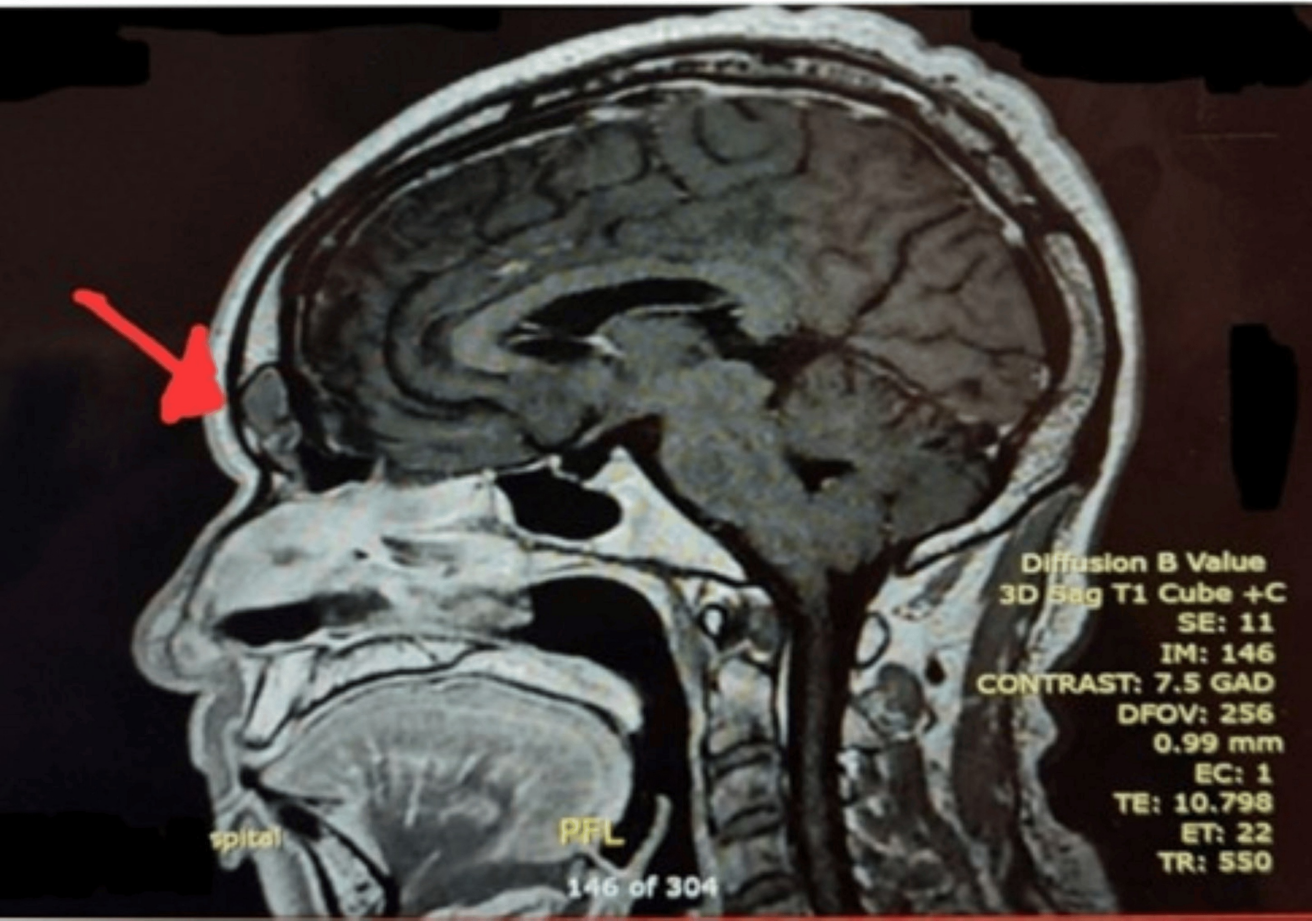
MRI brain (T1-weighted, sagittal view, post-contrast) showing a poorly defined calvarial lesion in the left frontal bone measuring approximately 34 mm with low signal intensity on T1-weighted images (arrow).
